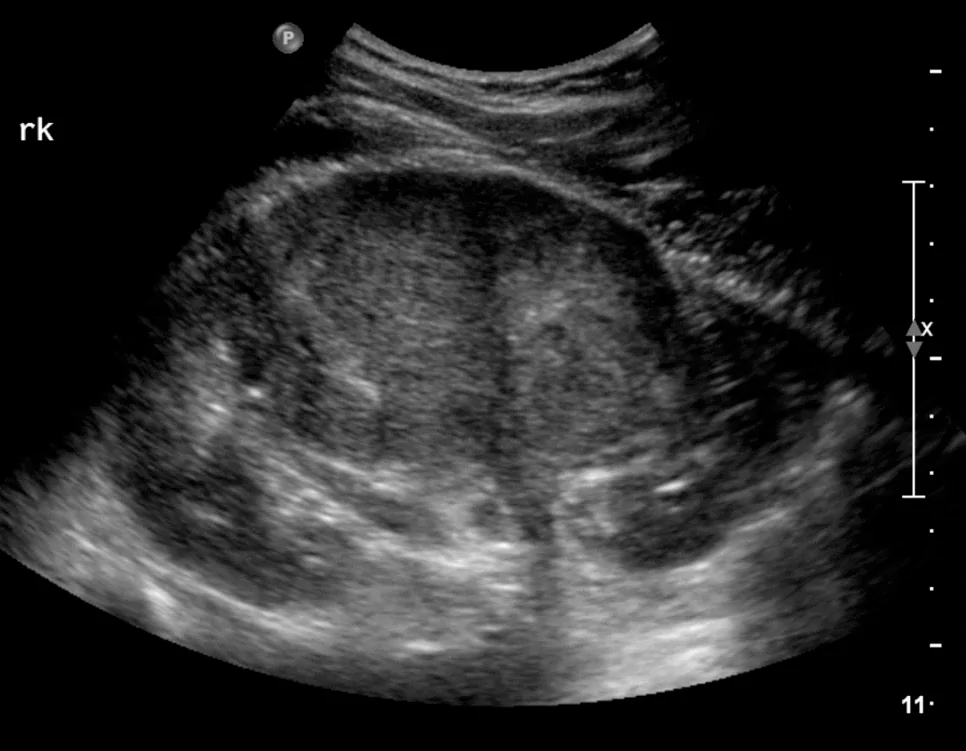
20-year-old Black male with sickle cell trait presenting with haematuria. Ultrasound scan showing an endophytic 8 cm mass in the interpolar region of the right kidney, compressing the collecting system
